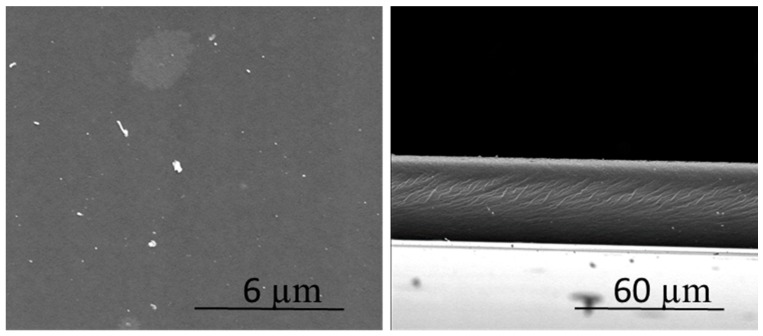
SEM images of a PIM with a composition of 50% CTA, 30% THTDPCl, and 20% NPOE. (Left) surface; (right) cross-section.
